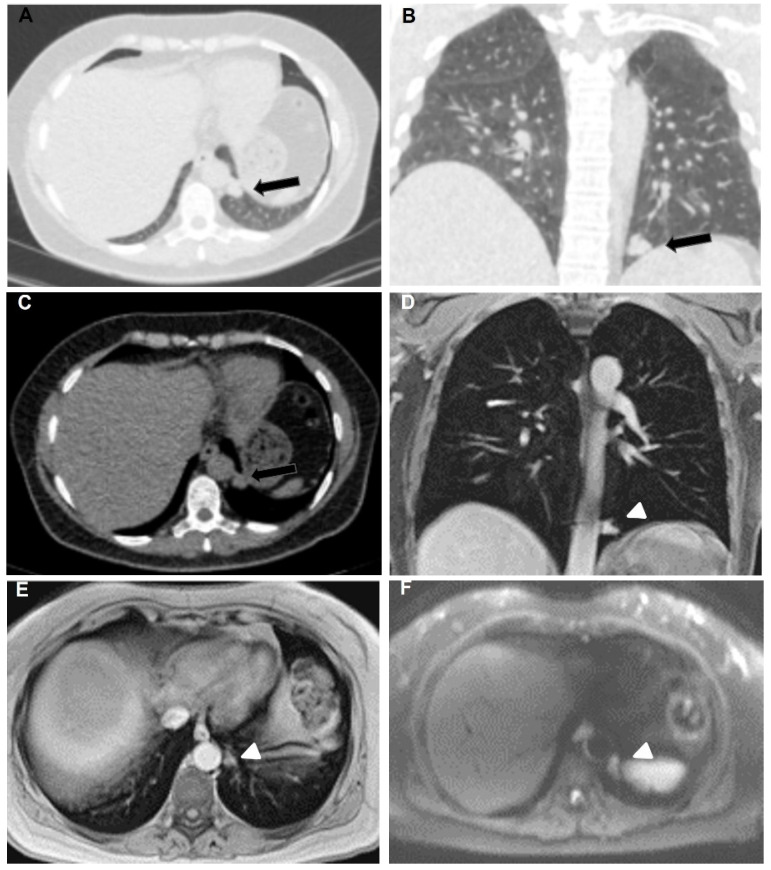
53-year-old female with breast adenocarcinoma. Axial (A) and coronal (B) lung window and axial (C) mediastinal window CT chest show solid, well-defined, lobulated, noncalcified pleural nodular lesion in the left hemithorax (black arrows). Coronal (D) and axial (E) Gd-enhanced T1-weighted chest MRI images feature the same nodule (arrowheads) as demonstrated in CT. DWI b800 axial chest MRI (F) shows diffusion restriction.
